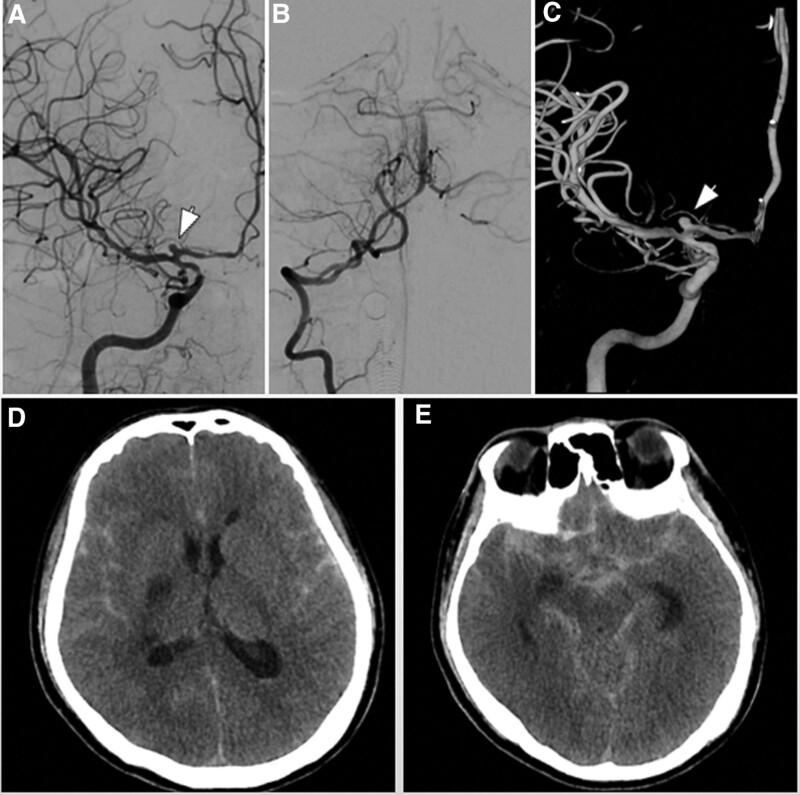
(A–C) Cerebral digital subtraction angiography showed a cystic aneurysm in the A1 segment of the right anterior cerebral artery with a size of approximately 2.8 × 2.9 mm and a neck of approximately 2.3 mm. (D–E) Repeat head CT showed extensive subarachnoid hemorrhage with intraventricular hemorrhage.
